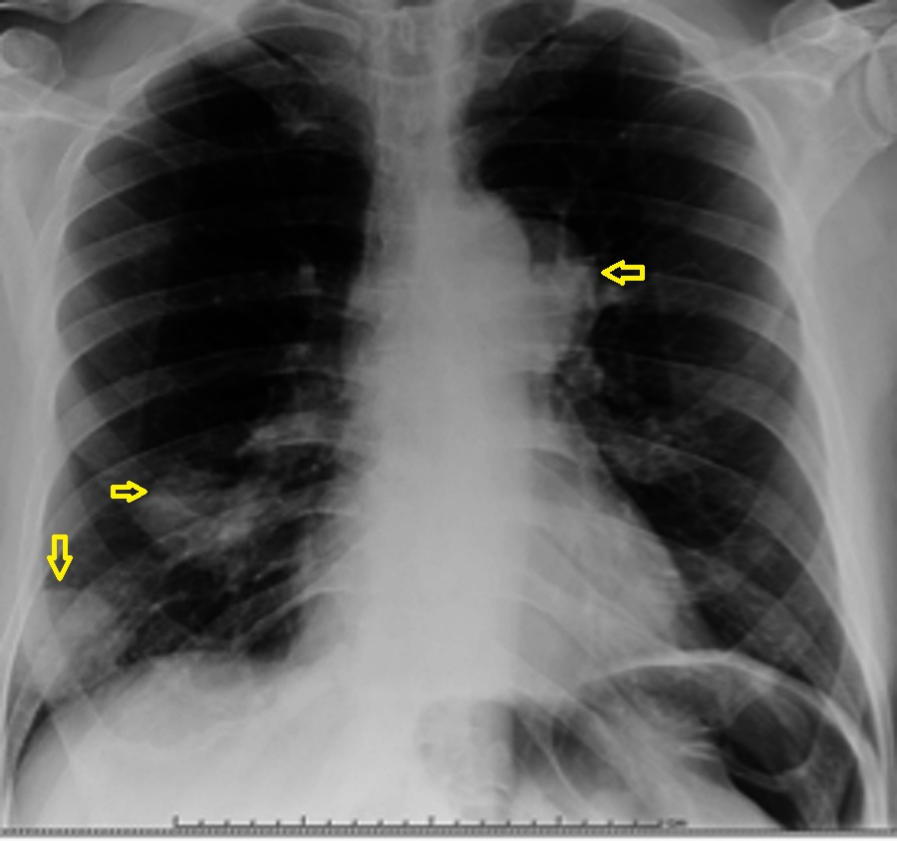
Chest X-ray showing multiple masses in the lung (arrows).
